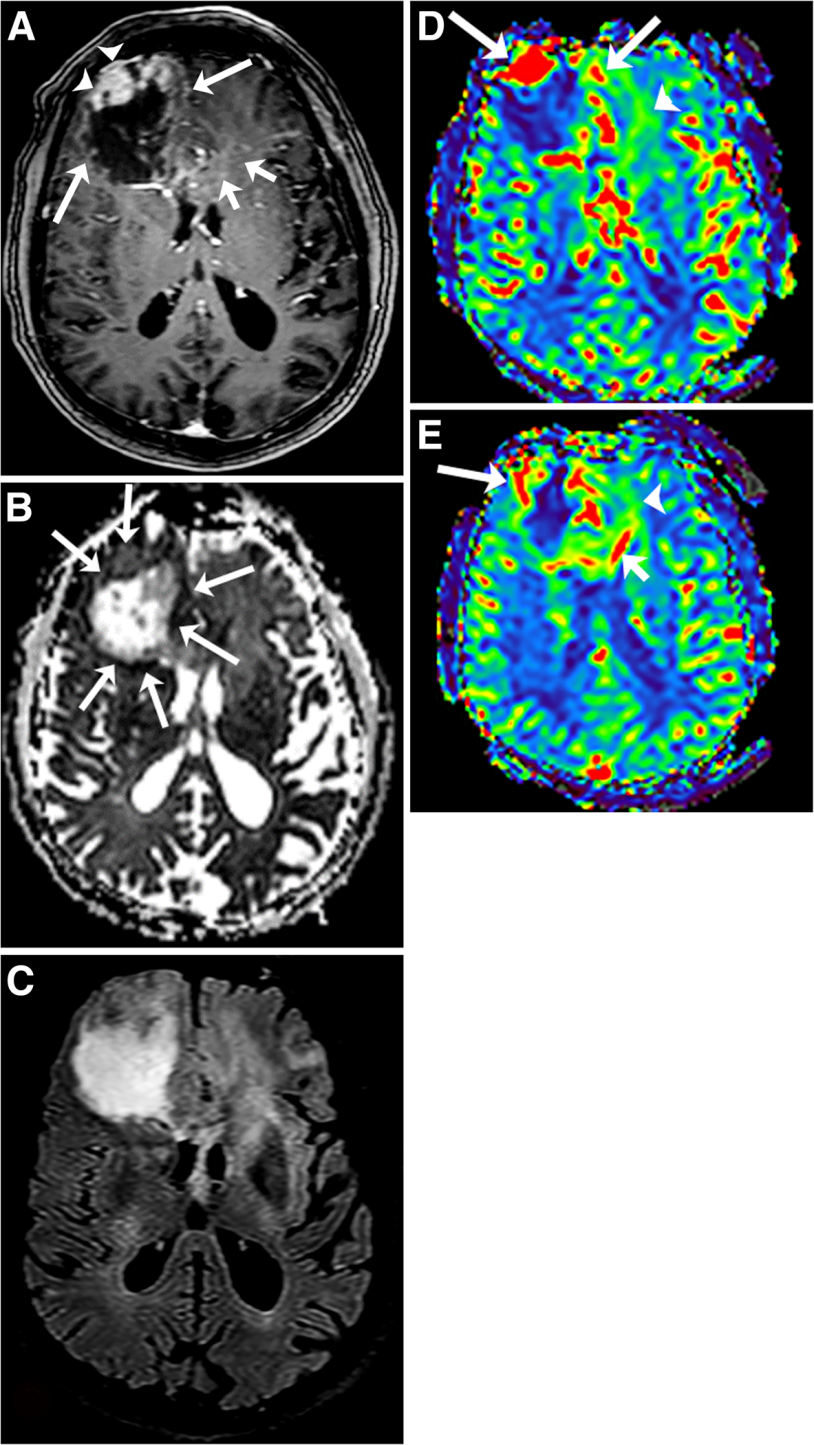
Glioblastoma. T1-weighted image with gadolinium (a) shows an irregular rim-enhancing mass with thick (arrowheads) and thin (long arrows) areas of enhancement in the right frontal lobe. The mass extends into the genu of the corpus callosum and left frontal lobe with minimal irregular enhancement (short arrows). ADC map (b) demonstrates predominant elevated ADC within the necrotic mass. The rim of the mass has minimal decreased ADC values (arrows). T2 FLAIR (c) shows the necrotic right frontal lobe mass, enlargement of the genu of the corpus callosum and diffuse mild T2 high signal abnormality in the left frontal lobe. On CBV map (d, e), there is associated increased perfusion in the rim of the mass (long arrows) in the right frontal lobe, corpus callosum (short arrow), and to a lesser degree in the left frontal lobe (arrowheads)
